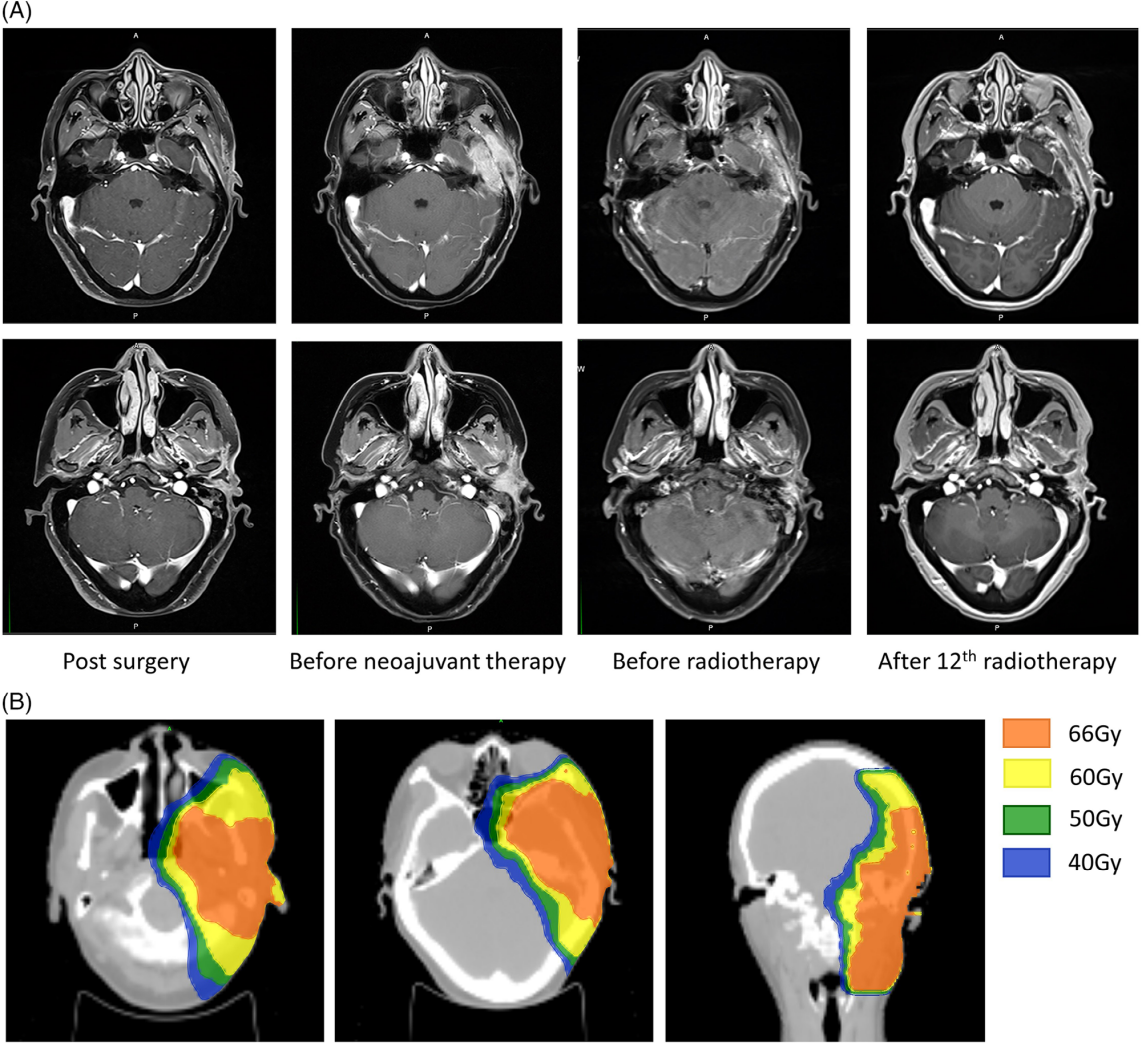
Representive magnetic resonance imaging during treatment (A) and isodose line of target volume images (B) of the patient.
